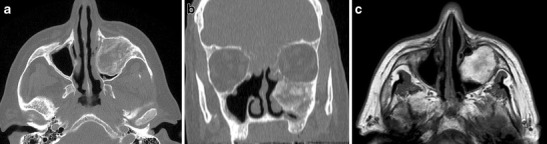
a) Axial and b) coronal CT images with c) TI-weighted axial MRI at the level of the maxillary sinuses in a 60-yr-old male with HPT-JT. The patient presented with symptoms of chronic sinusitis and CT imaging demonstrates an ossifying fibroma which arises from the maxilla and almost obliterates the left maxillary sinus. On MR the imaging features are those of a partially ossified expansile fatty soft tissue mass, areas of focal low signal on the T1 weighted sequence reflect the calcified osseous components of the ossifying fibroma. There is evidence of previous sinonasal surgery with wide bilateral medial antrostomies, middle turbinectomies, uncinectomies and ethmoidectomies. Opacification, suggestive of a mucocele, was noted in the anterior ethmoid and frontal sinuses
